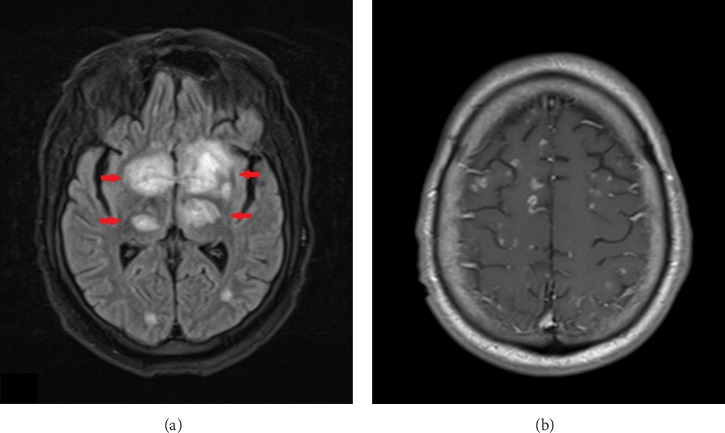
MRI of the brain without contrast shows an increase in both the number and size of the previously noted enhancing lesions throughout the brain parenchyma ((a) and (b)), affecting both the cerebral and cerebellar hemispheres as well as the brainstem. The largest lesions are located in the basal ganglia and thalami ((a) red arrows).
